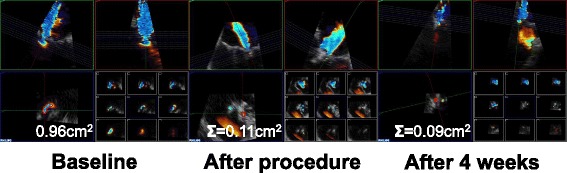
Vena contracta area determination by 3D–TEE is exemplarily shown for a DMR patient. Shown are MPR views at three time points. Each MPR view is based on one recorded data set and composed of a fourfold table. Top left (green box): midoesophageal long axis view. Top right (red): orthogonal plane to green box. Bottom left (blue): 3D–en face view to VCA (traced by red line). Bottom right: multislice representation of 9 evenly distributed slices parallel to 3D–en face view, used to find actual VCA plane. VCA was defined as the central laminar jet core (within red lines) as defined by current ASE guidelines [2]. Immediately after PMVR the residual regurgitant jet was split into at least two. VCA of each jet was determined separately and summed up. After PMVR, measurement of one jet is exemplarily shown. VCA: vena contracta area. DMR: degenerative mitral regurgitation. MR: mitral regurgitation. Σ: two residual jets were summed up. ASE: American Society of Echocardiography
